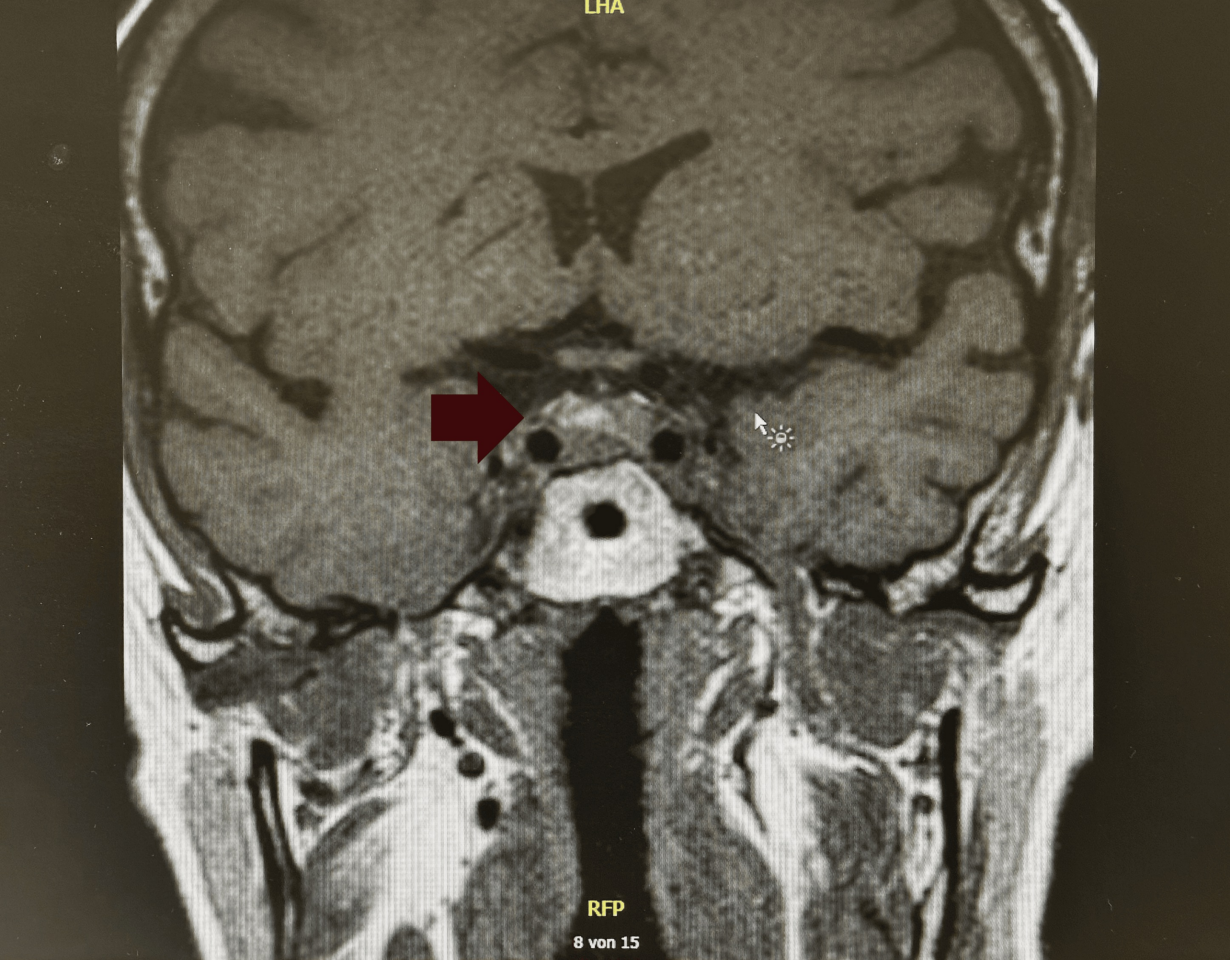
Coronal T1-weighted MRI showing a GH-secreting pituitary macroadenoma (10 × 14 × 9 mm) occupying the sella turcica (red arrow). Preoperative image showing the larger of two intrasellar lesions (red arrow) expanding the sella turcica, consistent with a macroadenoma. At the time of imaging, the patient’s ACTH level was markedly elevated (1,489 pg/mL), supporting a diagnosis of primary adrenal insufficiency (Addison’s disease) rather than pituitary dysfunction or compression. The tumor was later confirmed to be GH-secreting, not ACTH-secreting. MRI: magnetic resonance imaging; GH: growth hormone; ACTH: adrenocorticotropic hormone
